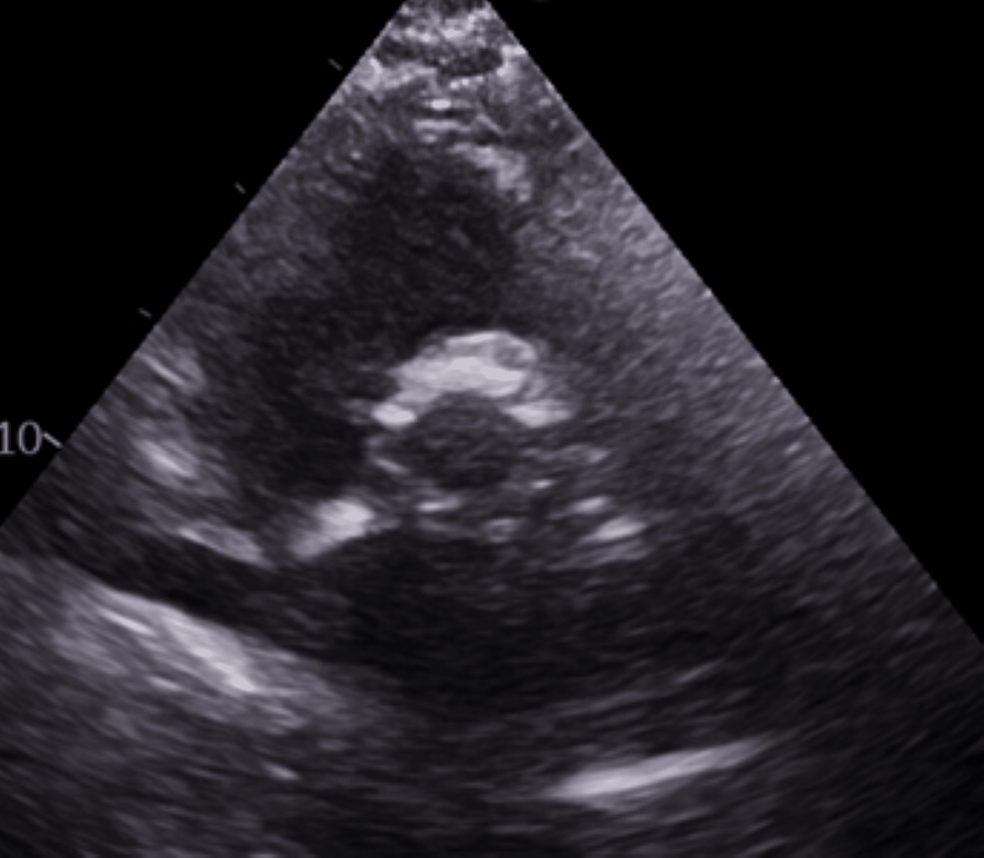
Six Weeks Post TAVR. Thrombosed Valve while on Apixaban Ultrasound parasternal short-axis view showing thickening of bioprosthetic valve leaflets. TAVR: transcatheter aortic valve replacement
